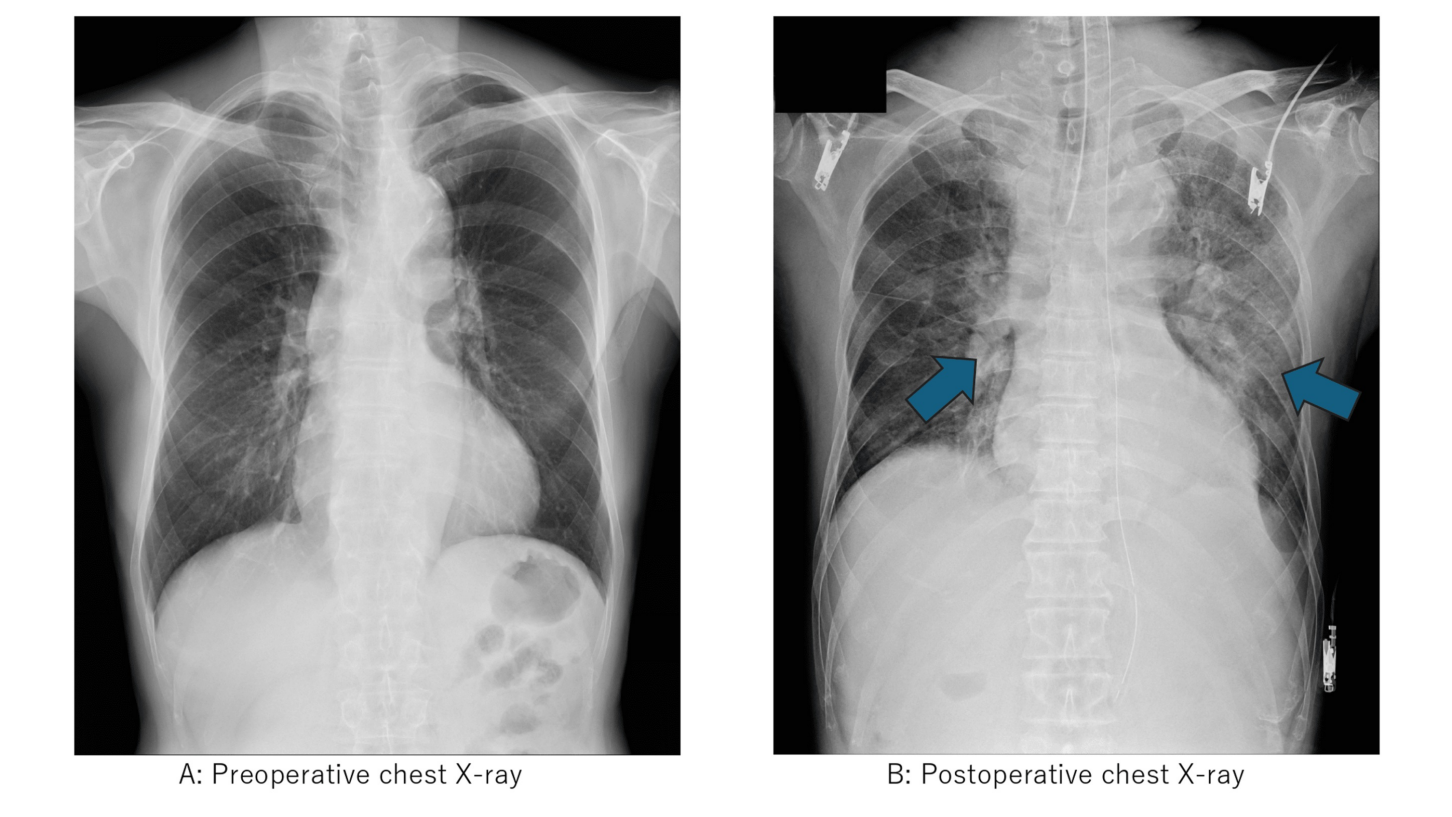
Chest radiographs (A) Preoperative posteroanterior chest radiograph: clear lung fields with normal cardiac silhouette and pulmonary vascular markings. (B) Postoperative (six hours post incident): bilateral symmetric pulmonary edema with perihilar and peripheral alveolar infiltrates consistent with transfusion-related acute lung injury (TRALI), taken when advanced imaging became available in the high-dependency unit. Blue arrows indicate areas of bilateral pulmonary infiltrates characteristic of TRALI. Note the absence of cardiomegaly or pleural effusions, supporting a non-cardiogenic etiology.
